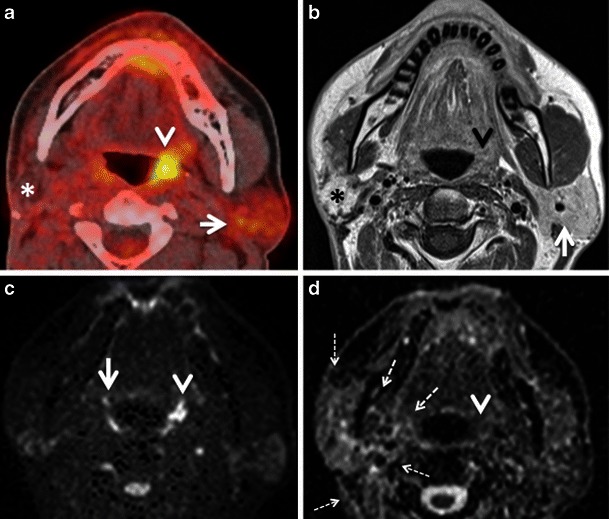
a Axial PET/CT image demonstrates asymmetrical FDG uptake of the tonsillar fossae (*arrowhead*) and parotid glands (increased uptake on the left, *arrow*) in a patient previously irradiated for SCC of the right base of the tongue with sparing of the left side. Note increased FDG uptake of the non-irradiated left parotid gland (*arrow*) and fatty infiltration of the irradiated right parotid gland (*asterisk*). b Corresponding axial contrast-enhanced T1-weighted image shows no pathological findings in the left tonsillar fossa (*arrowhead*) and left parotid gland (*arrow*). Note atrophy and fatty infiltration of the right parotid gland (*asterisk*). c Corresponding *b*1,000 DW MR image reveals physiological hyperintensity in the tonsillar fossae more pronounced on left (*arrowhead*) compared with the right (*arrow*) due to normal lymphoid tissue on the left and atrophy of lymphoid tissue on the right. d Corresponding ADC map showing physiologically low ADC values on the left (*arrowhead*) due to normal lymphoid tissue. *Dashed arrows* point at radiation port on the right
